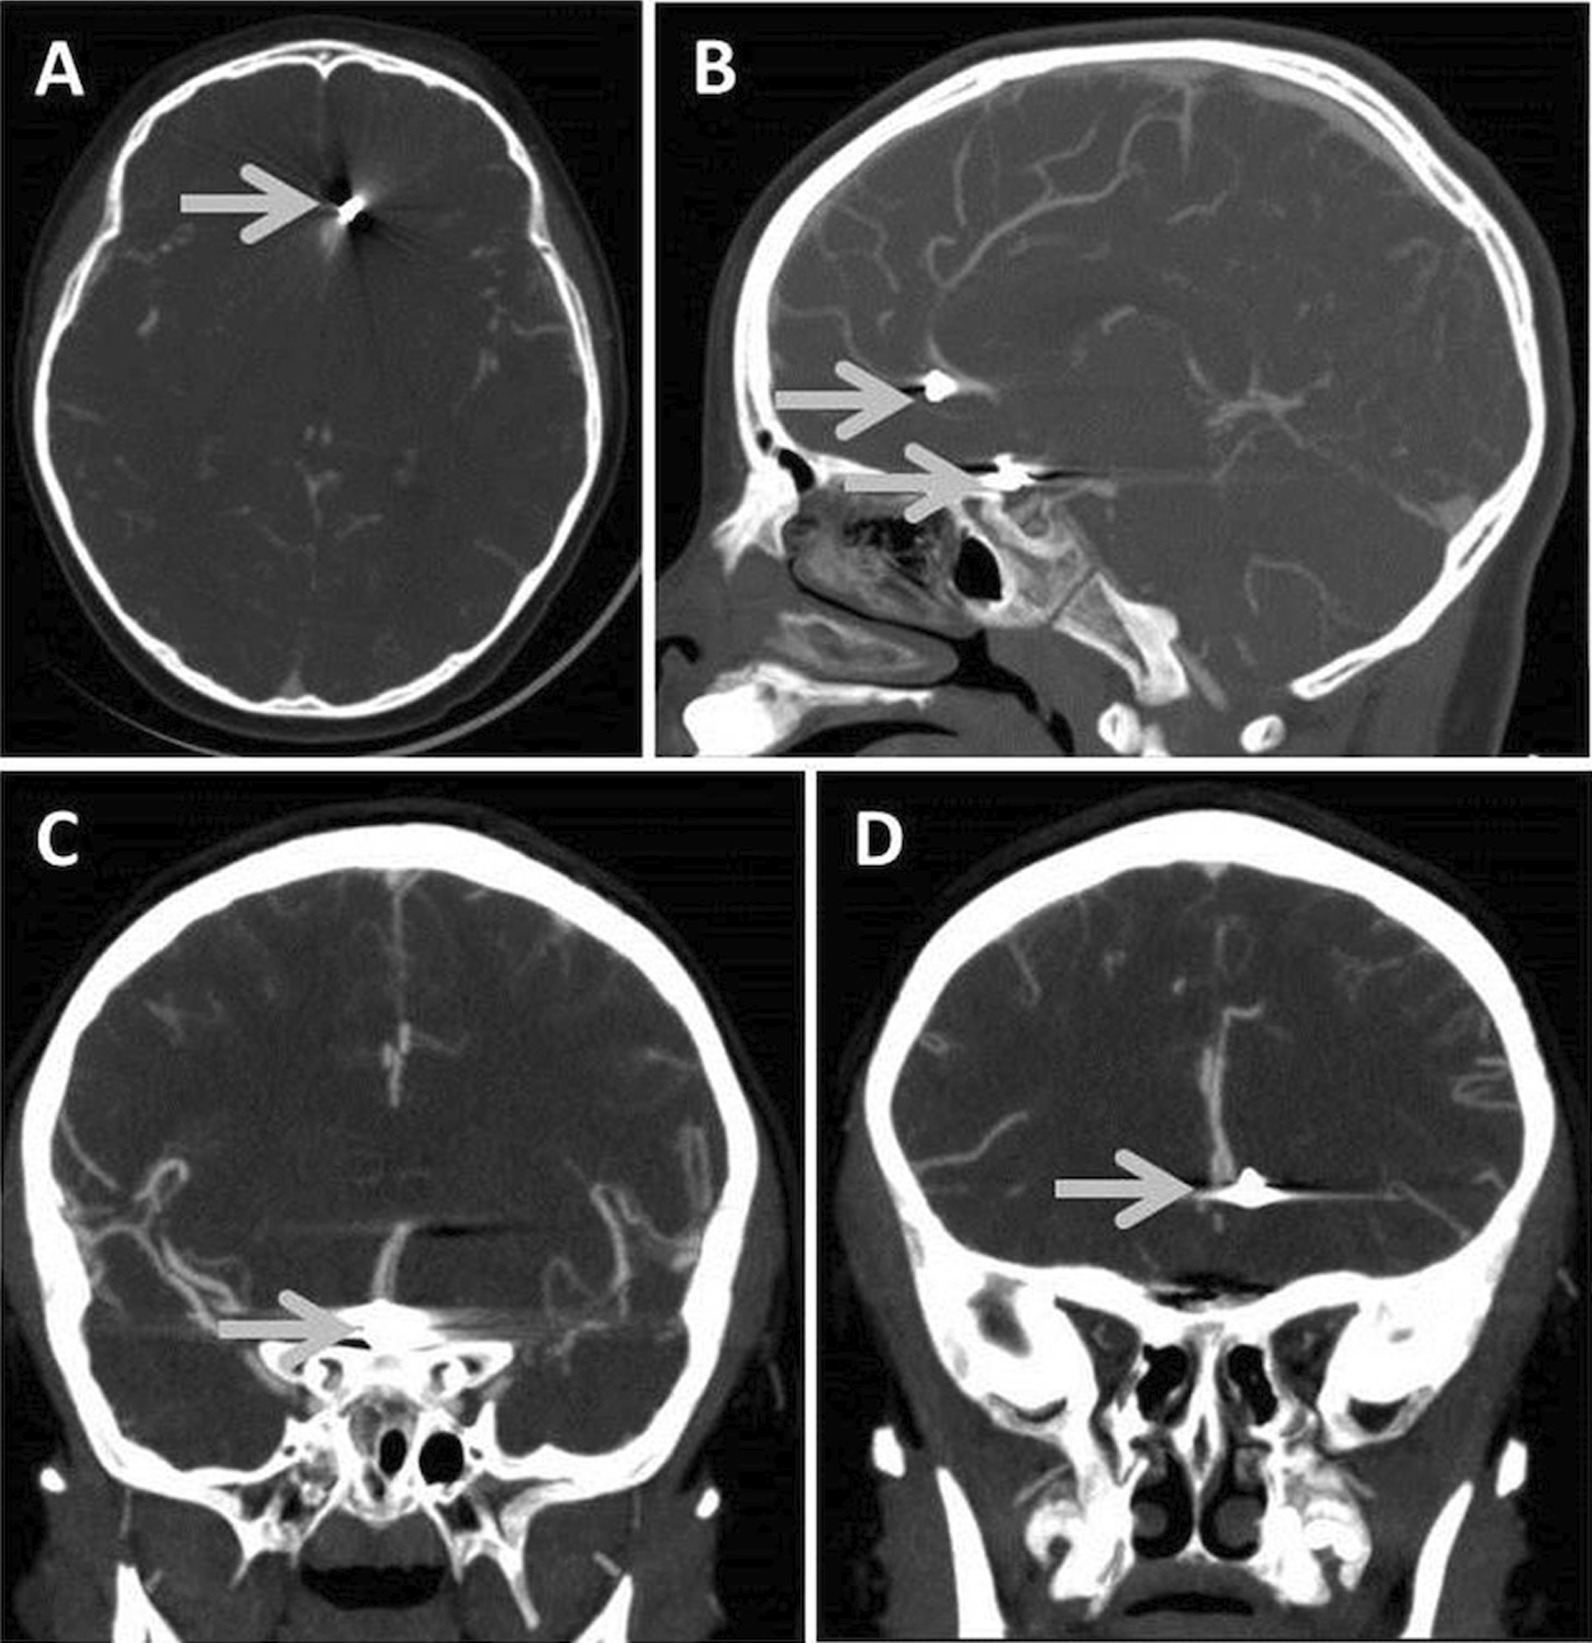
Axial (A), sagittal (B), and coronal (C, D) views of CTA study, confirming the location of two intracranial, metallic shrapnel fragments from a BB pellet gun injury (shrapnel demarcated by gray arrows)
